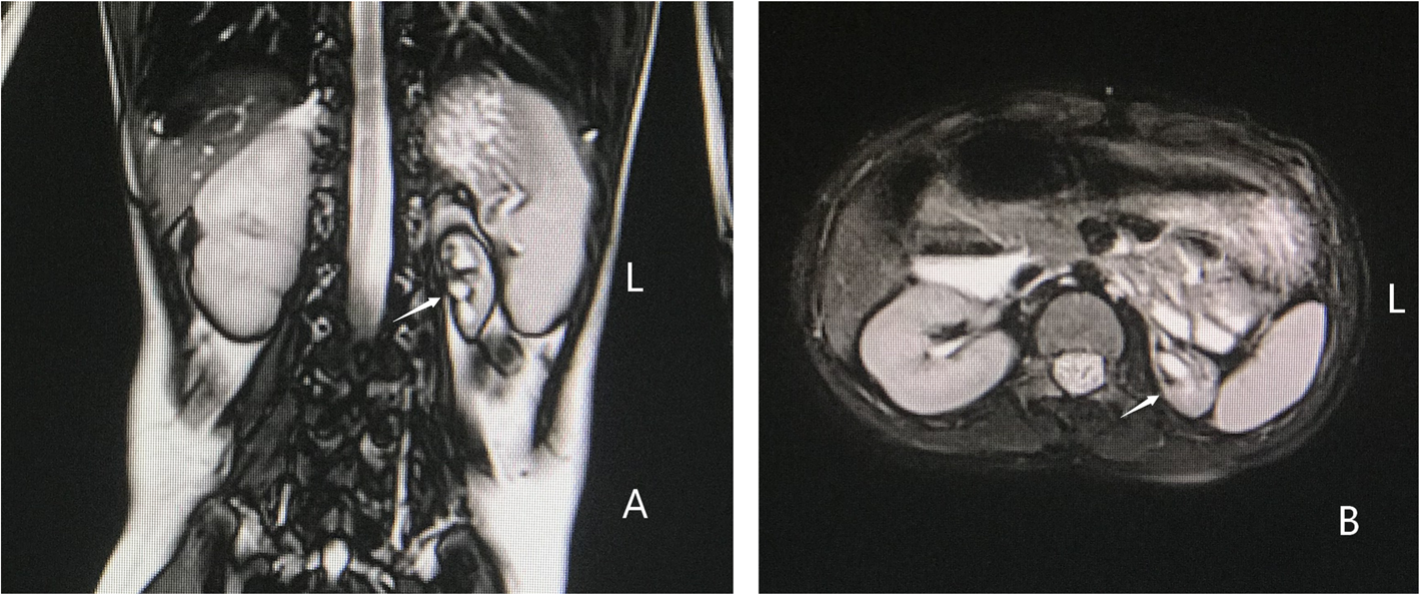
Renal MRI of the patient, viewing the kidney in the coronal plane (a) and the transverse plane (b). The left kidney was small in size; the boundary between cortex and medulla was not clear; renal pelvis and calyces dilation was detected. The right kidney was normal in shape and size
